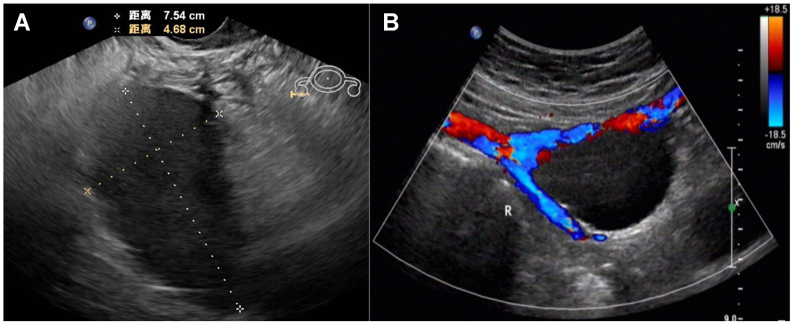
(A) Color Doppler ultrasound examination demonstrates a 75.4 mm × 46.8 mm anechoic mass adjacent to the right iliac vessels. (B) Corresponding Doppler image confirms the absence of internal flow signals within the lesion.
